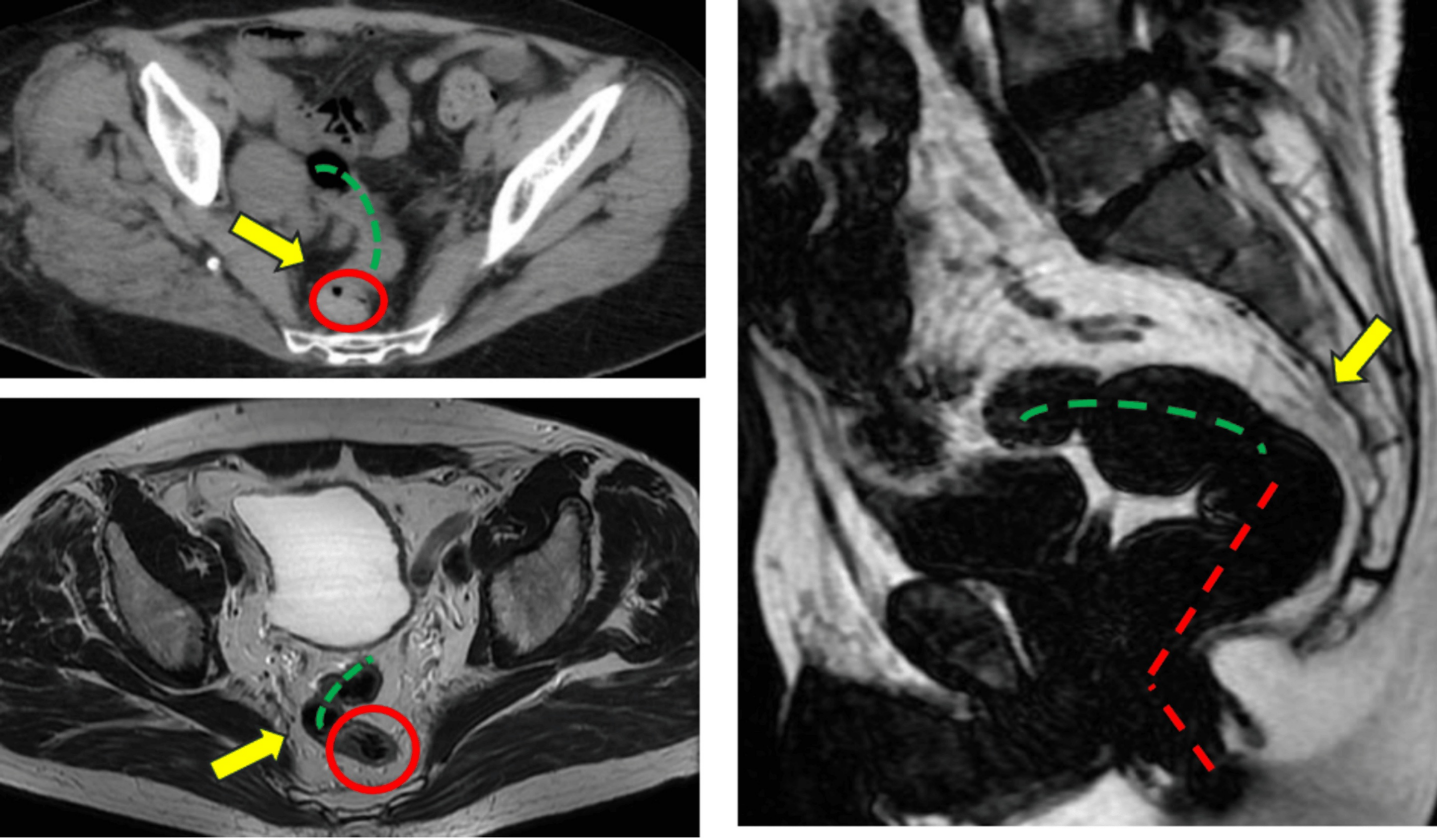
CT and MRI axial view and MRI sagittal view: sigmoid take-off, yellow arrow; rectum, red circle/dotted line; and sigmoid colon, green dotted line. Images are from the authors' patients and are not published elsewhere.
